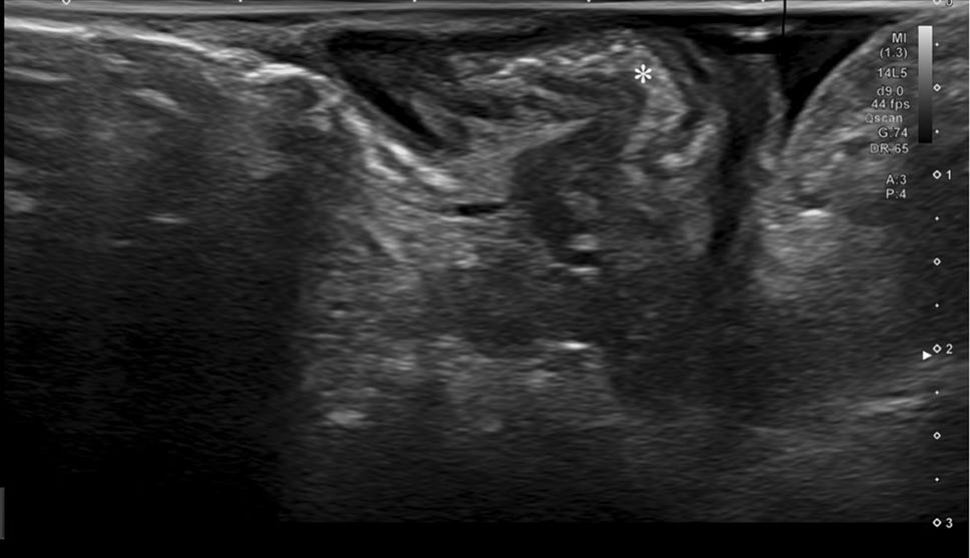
Skip lesion detected on intraoperative ultrasound. *Thickening of the mucosa and submucosa of the small bowel
